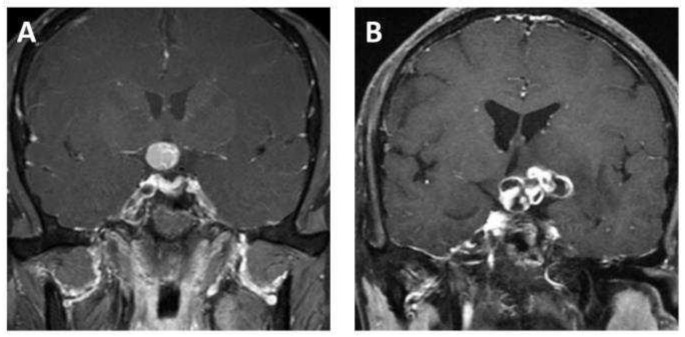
Representative contrast-enhanced T1-weighted MR images of (A) adamantinomatous craniopharyngioma and (B) papillary craniopharyngioma.
